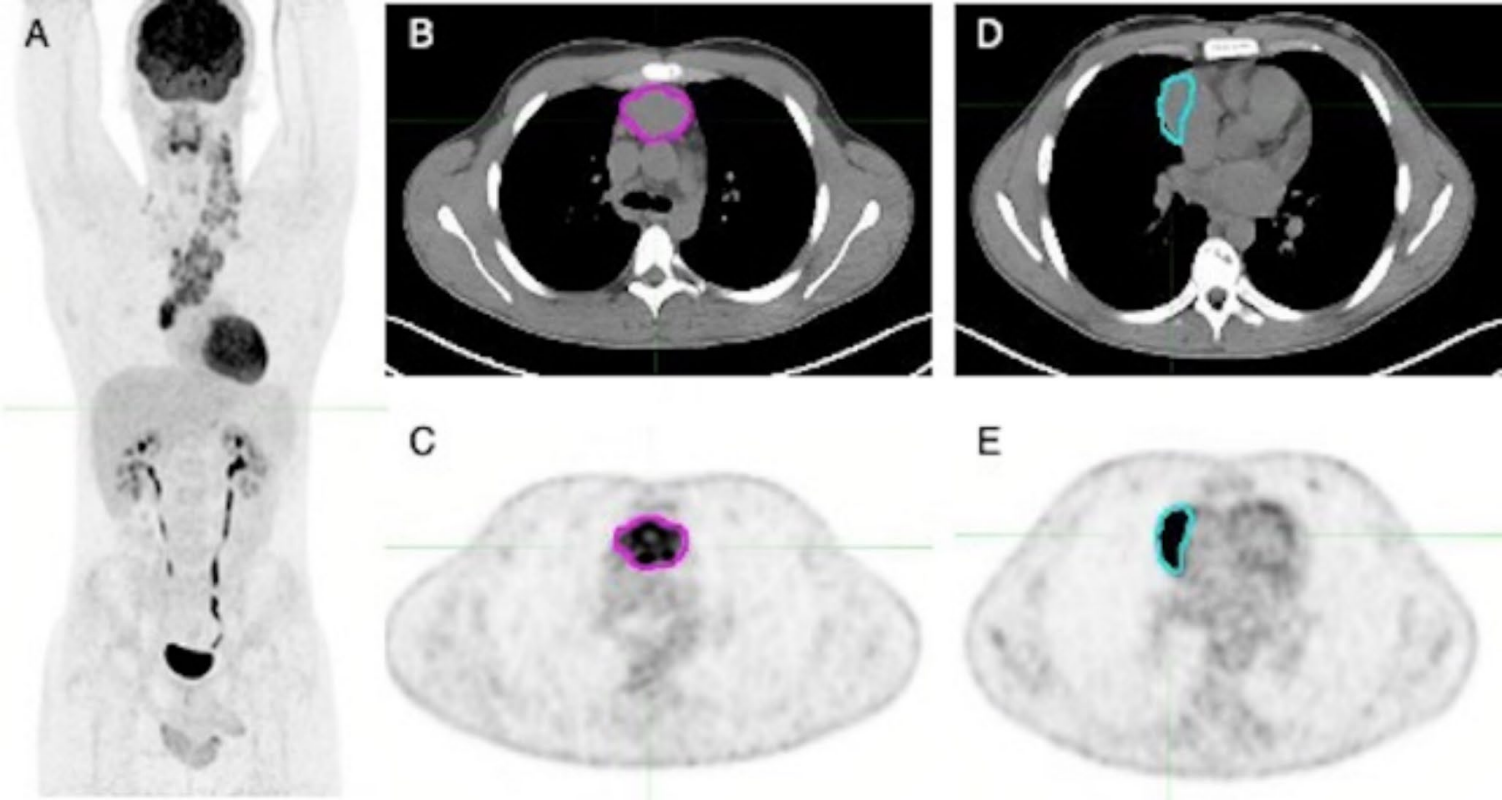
Target lesion contouring with PET_Edge_ tool from MIM Encore Software (version 7.0.5 MIM Encore Software Inc., Cleveland, OH). (A) Maximum-intensity projection of an 18-year-old male patient with Stage IIA nodular sclerosing Hodgkin’s Lymphoma. Axial CT (B) and PET (C) views of Lesion_A (pink contoured VOI, D_max_ = 4.33 cm). Axial CT (D) and PET (E) views of Lesion_B (cyan contoured VOI, SUV_max_ = 11.81)
